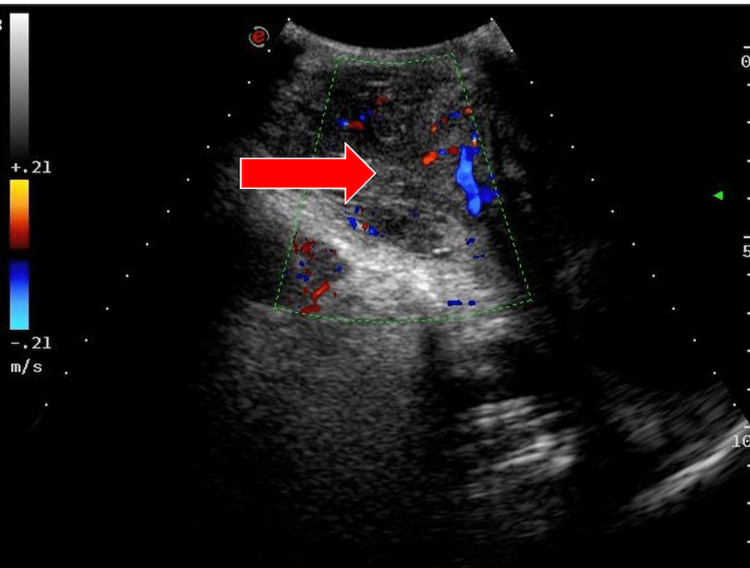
Ultrasound: scrotum - image 3 The image demonstrates increased and disorganized vascularity on color Doppler within the mass lesion
